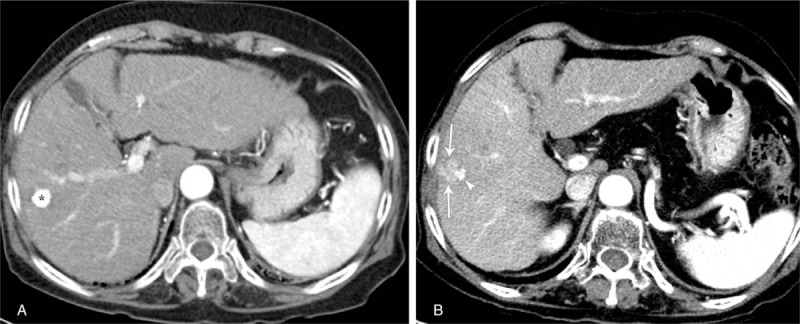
(A) Contrast-enhanced axial arterial phase CT image shows a compact lipiodolized lesion (asterisk) treated with TACE for a small HCC in the right hepatic lobe. (B) Fourteen-month follow-up contrast-enhanced axial arterial phase CT image depicts arterial enhancement in a recurrent viable HCC (arrows) around a contracted lipiodolized lesion (arrowhead) in the right hepatic lobe. CT = computed tomography, HCC = hepatocellular carcinoma, TACE = transarterial chemoembolization.
